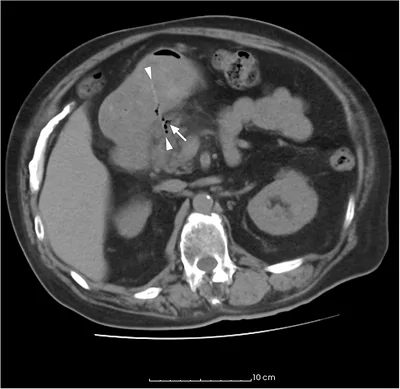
Non‐contrast computed tomography (CT) image obtained at presentation, showing a linear foreign body penetrating from the posterior wall of the lower body of the stomach into the head of the pancreas. The two arrowheads indicate the ends of the foreign body, and the arrow indicates the free air adjacent to it. Inflammatory fat stranding is seen around the foreign body. Window width, 350 HU; window level, 30 HU.
